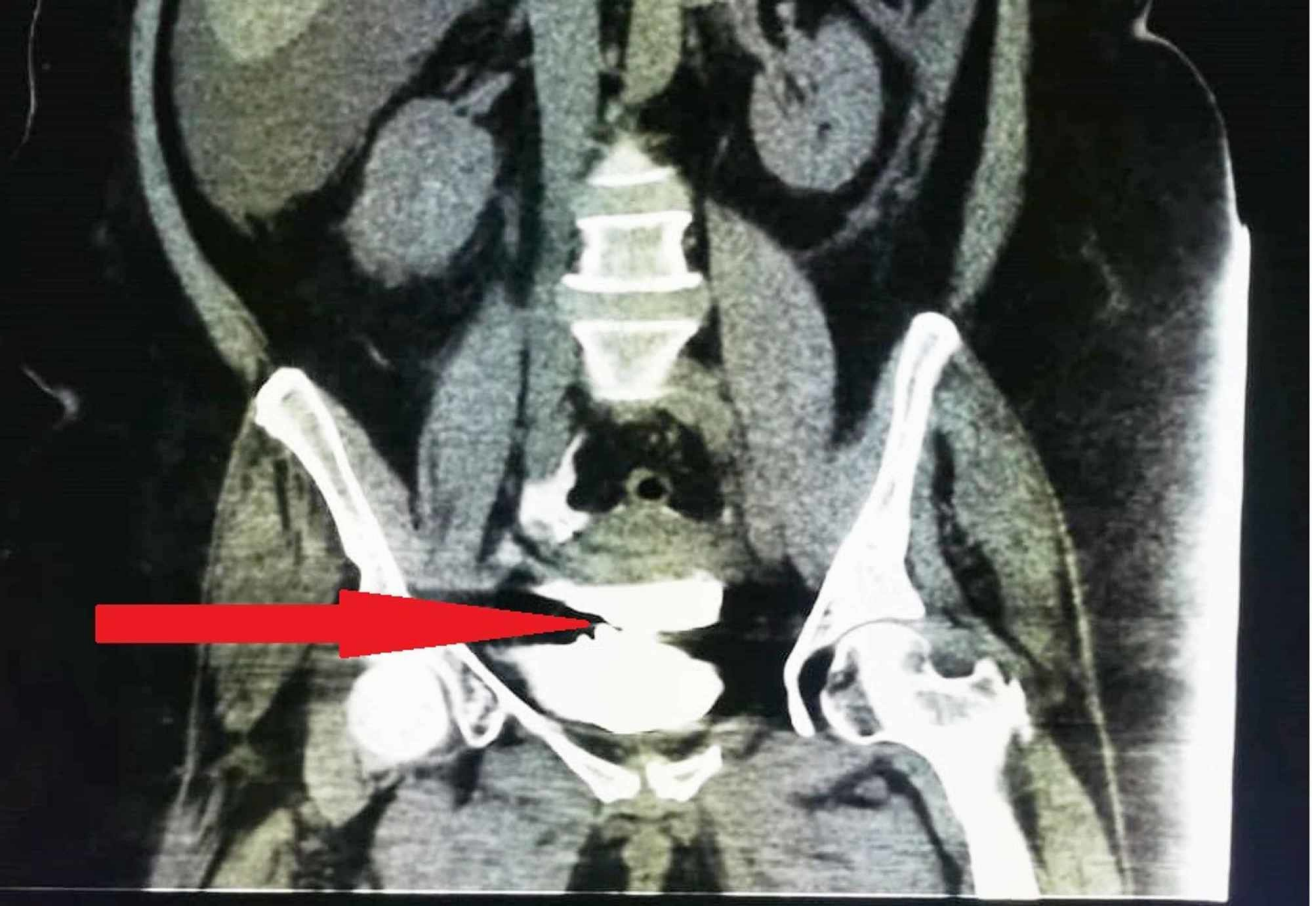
CT cystogram showing a defect in the dome of the urinary bladder with extravasation of contrast to intraperitoneal space
